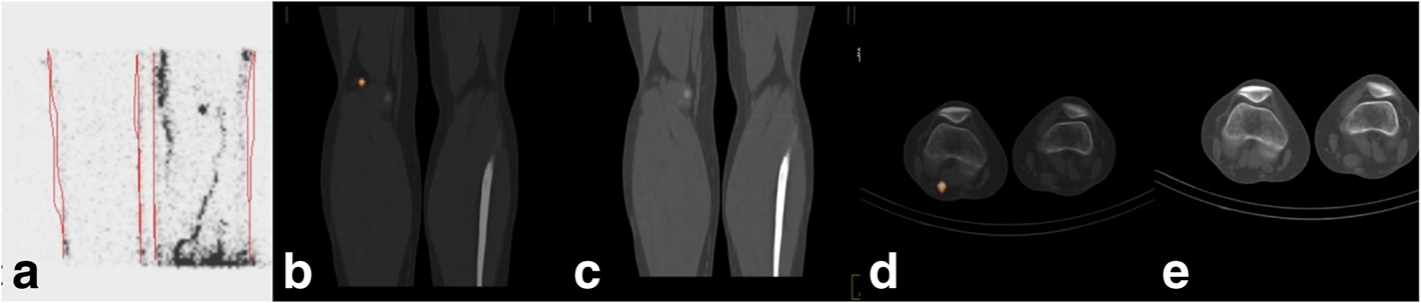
SPECT/CT. Patient with a malignant melanoma on the right foot. (a) Lymphoscintigraphy of the right popliteal region. (b) SPECT/CT in the sagital plane of the popliteal region with one SLN. (c) Low-dose CT in the sagittal plane of the popliteal region. (d) SPECT/CT in axial plane of the popliteal region with one SLN. (e) Low-dose CT in the axial plane of the popliteal region
